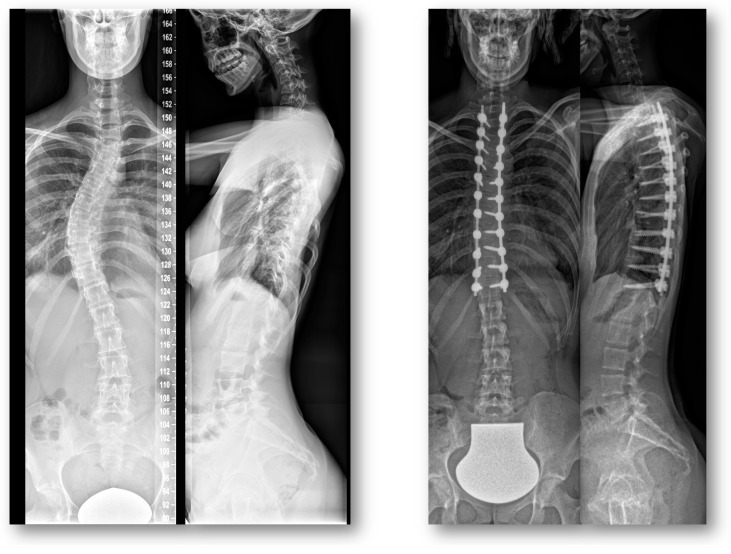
Pre-operative and post-operative coronal and lateral radiographs of study patients with adolescent idiopathic scoliosis and posterior fusion.
